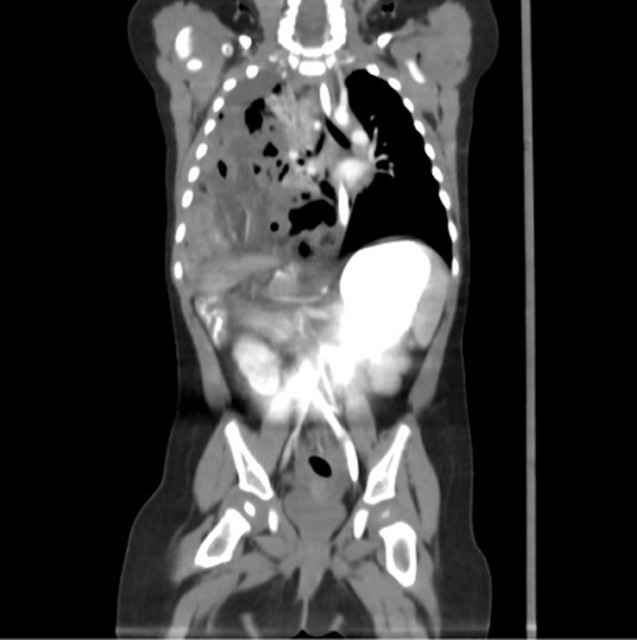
coronary axis CT: the right chest cavity almost completely occupied by loops of the gastrointestinal tract and intrathoracic projection of the mesenteric vessels
